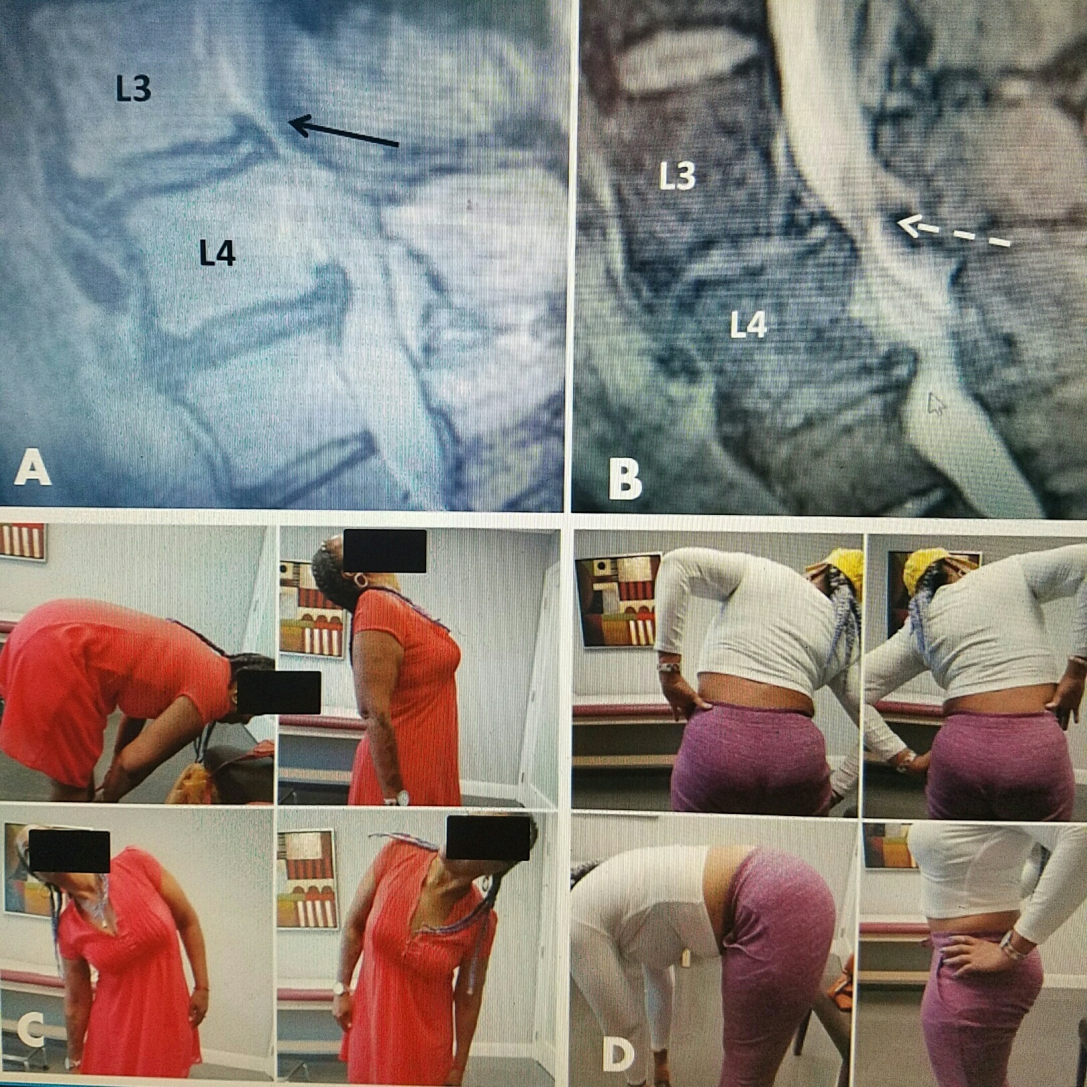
Patient movement after radiofrequency treatment for stenosis A: 58-year-old female with marked L3-4 stenosis (black arrow) with anterolisthesis at L3-4 and a small superior L4-5 disc extrusion. B: Post-treatment MRI follow-up at three months shows reduction in posterior ligamentous stenosis (white dashed arrow). C: Patient movement at three weeks post RF demonstrating full flexion, extension, and lateral bending. D: Patient continues with full normal movement six months post RF. MRI: Magnetic resonance imaging; RF: Radiofrequency.
